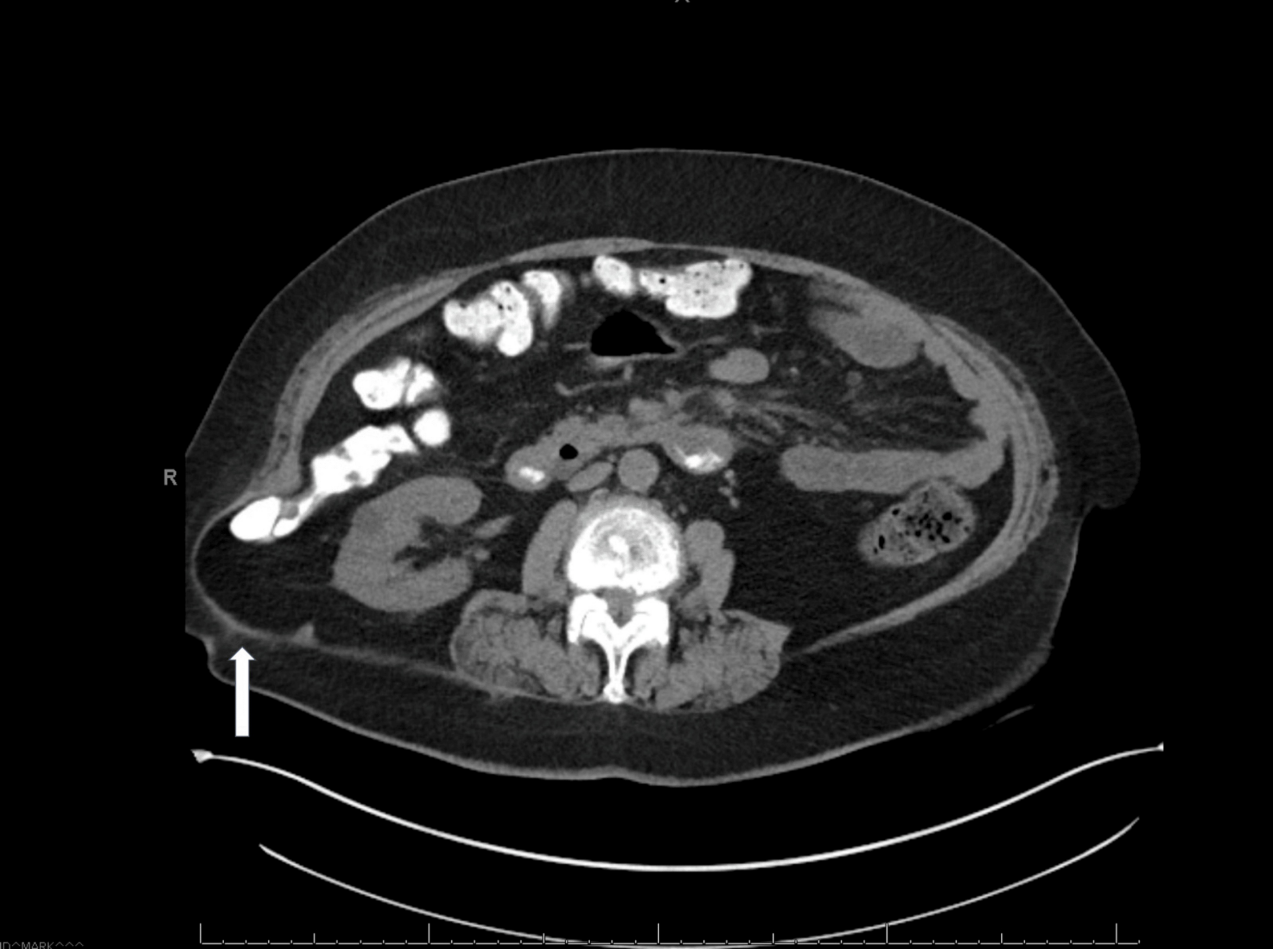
CT scan of the abdomen showing a hernia in the right posterior lumbar region CT, computed tomography
